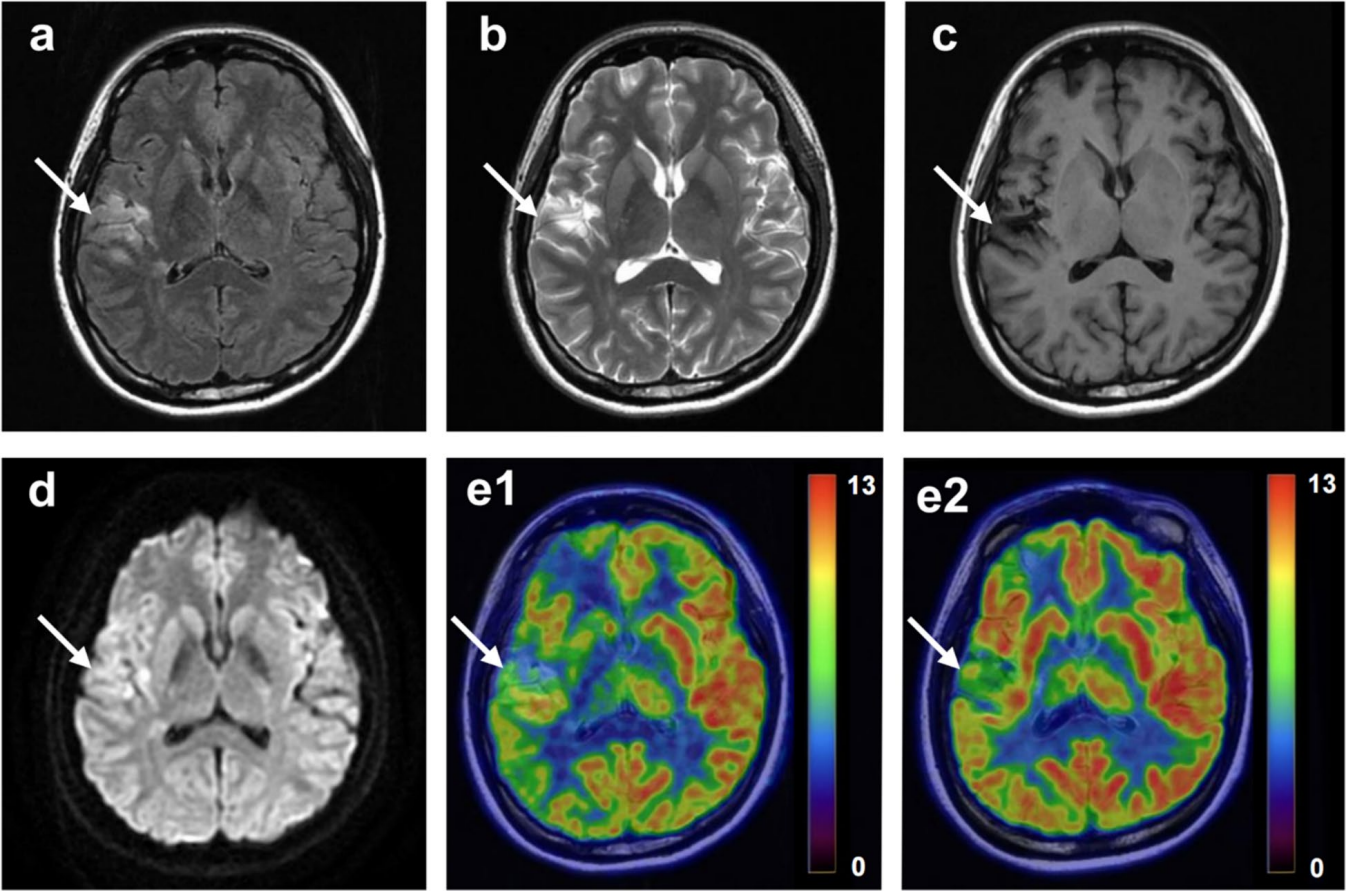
Example of a participate with ischemic cerebrovascular disease. A 24-year-old woman with ischemia in the right hemisphere. The NIHSS and mRS scores improved from 5 and 4 before follow-up to 1 and 2, respectively. On the T1 (a), T2 (b), T2-FLAIR (c), and DWI (d), the infarction can be seen in the right hemisphere. On the [^18^F]FDG PET-MR in the pre-follow-up period (e1), the metabolic impairment can be seen in the peri-infarct area. On the [^18^F]FDG PET-MR in the post-follow-up period (e2), the hypometabolic state of the infarct surrounding areas in the right cerebral hemisphere exhibited an improvement from the previous state
